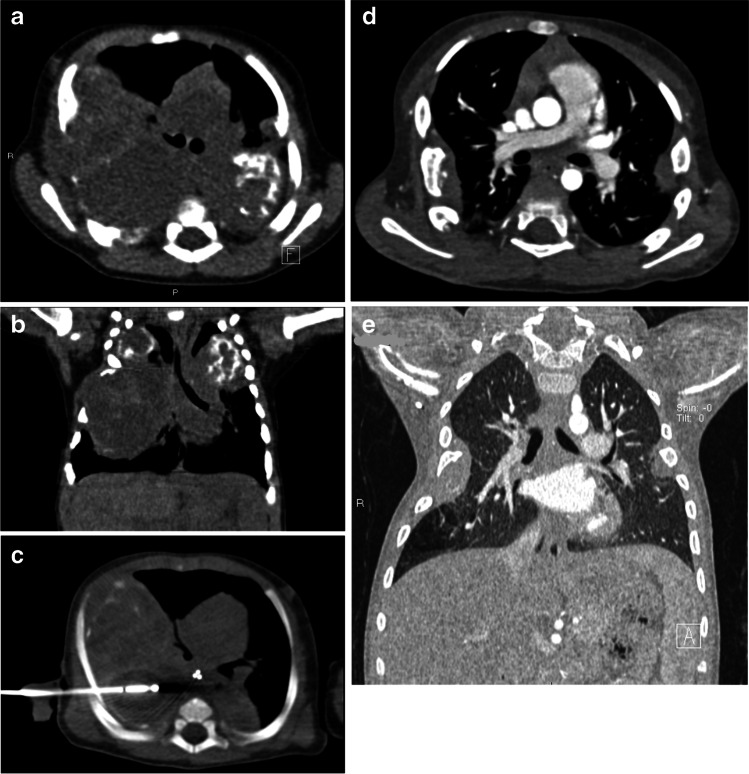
Mesenchymal hamartomas in a 4-month-old boy (patient 5) who was treated with cryoablation followed by radiofrequency thermoablation. He presented with acute respiratory distress and chest radiograph revealed bilateral masses of the thoracic wall. a, b Contrast-enhanced CT shows inhomogeneously hypodense oval lesions, with calcifications, arising from 4th–5th–6th right ribs and 4th left rib (a, axial; b, coronal). c He had cryoablation (axial CT image). Initial treatment was unsuccessful (tumor development and persistent dyspnea were seen), and he later underwent radiofrequency thermoablation. d, e Results were very good at 2-year follow-up, confirmed by contrast-enhanced axial (d) and coronal (e) CT images
